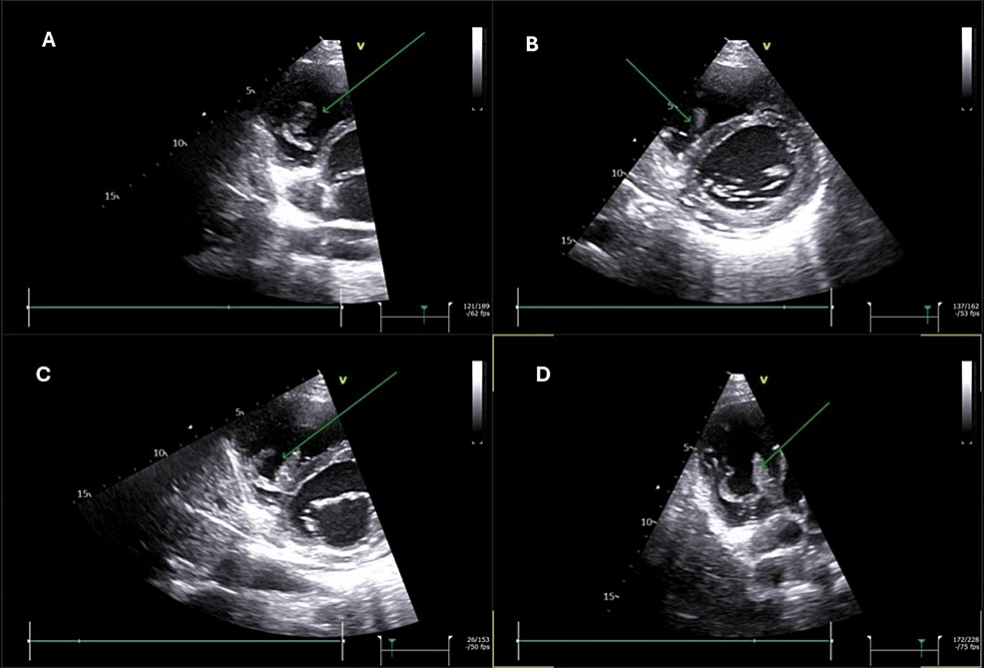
Case 1: Follow-up echocardiography. The green arrow shows a new thrombus curling in the right ventricle. Panel A: Short-axis view, mitral-valve level, zoomed-in view. Panel B: Short-axis view, papillary-muscle level. Panel C: Short-axis view, mitral-valve level. Panel D: Four-chamber dedicated right-ventricular view.
